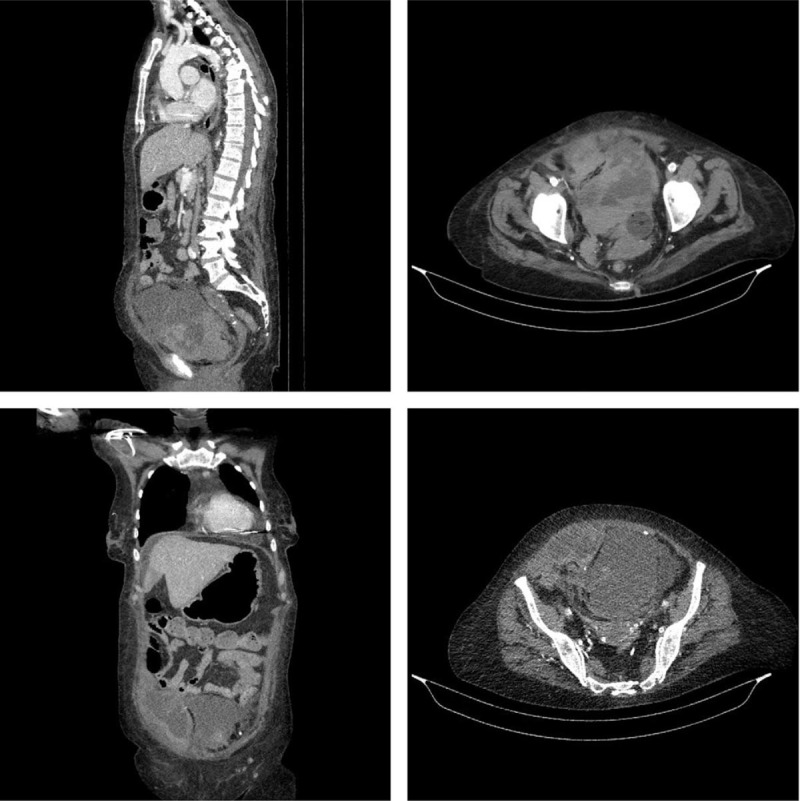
Multidetector computed tomography of abdomen and pelvis with intravenous contrast application, reveals hematoma in the right rectus abdominal muscle and large preperitoneal hematoma in Retzius space, displacing the bladder dorsally.
